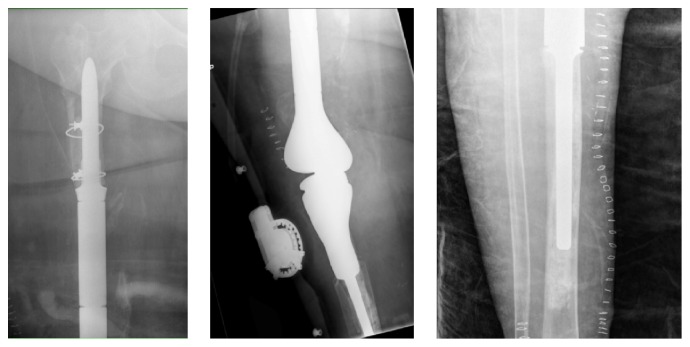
Postoperative radiographs of large endoprosthesis reconstruction.
